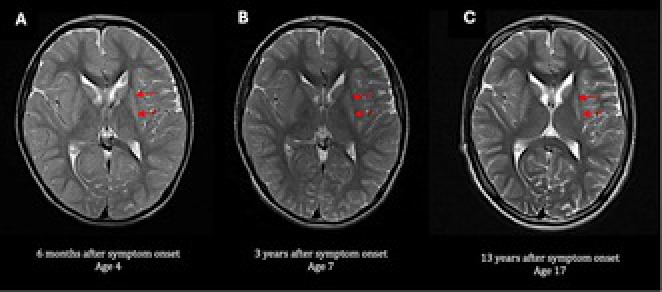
FIGURE 1 Longitudinal MRI evolution of bilateral putaminal involvement in DYT‐PRKRA.
